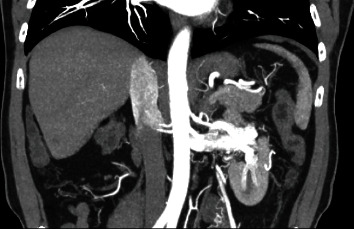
Coronal CT arterial phase showing two left renal arteries and early venous filling of contrast in renal vein and vena cava concerning for AVM in addition to multiple collateral vessels.
